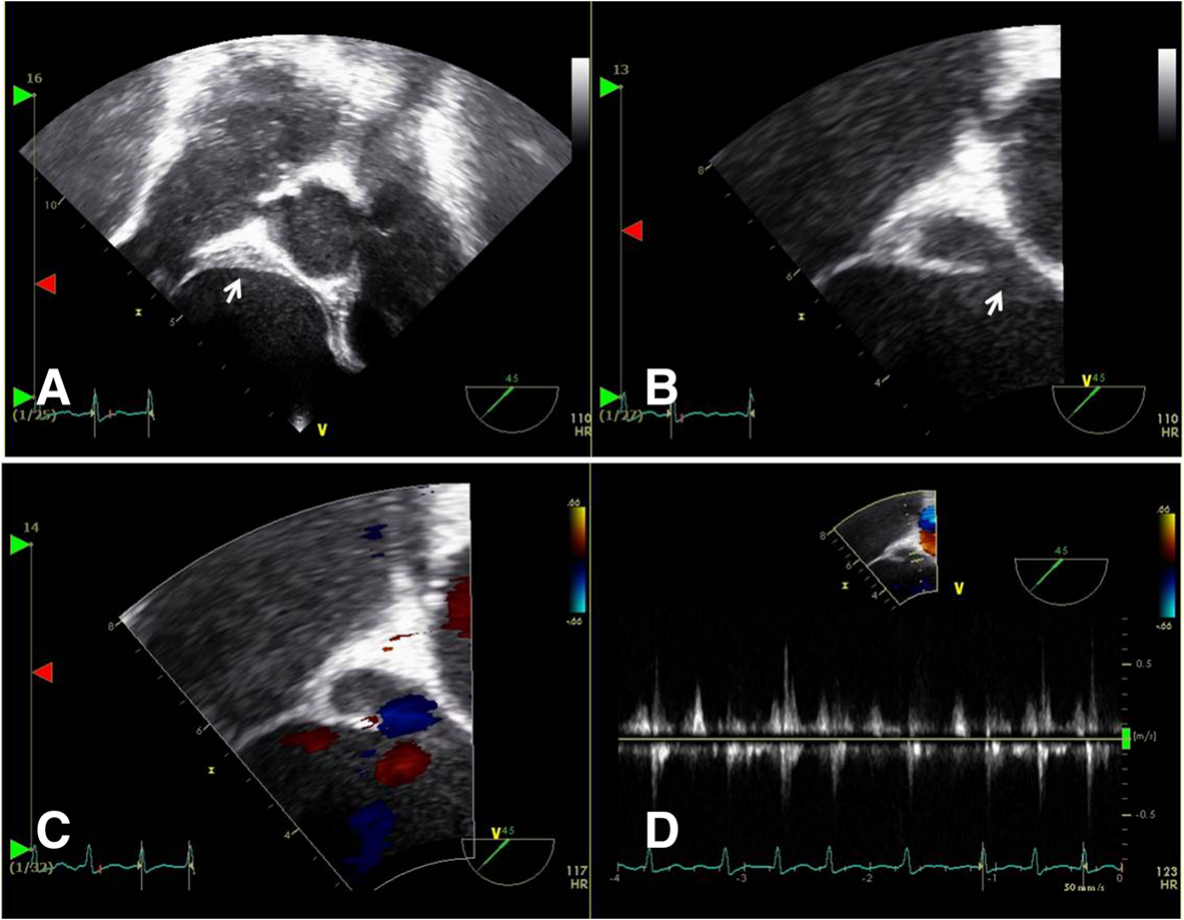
Transesophageal echocardiography shows a thickened nonhomogeneous area with echo-dense appearance around the aortic root (a, *white arrow*), as well as discontinuous endocardial tissue (b, *white arrow*) with flow communication detected by color and pulsed wave Doppler ultrasound (c and d)
